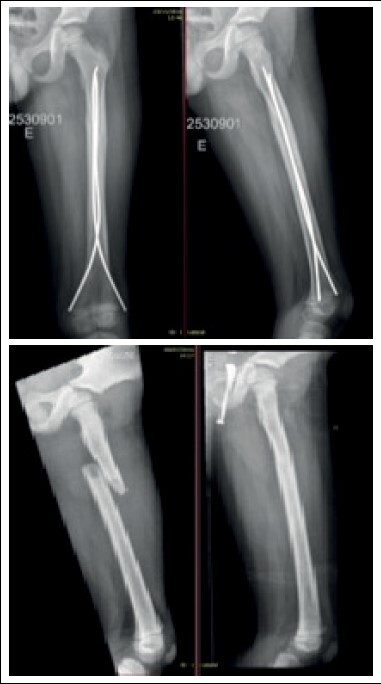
Preoperative and postoperative radiographs (4 months after fracture).
